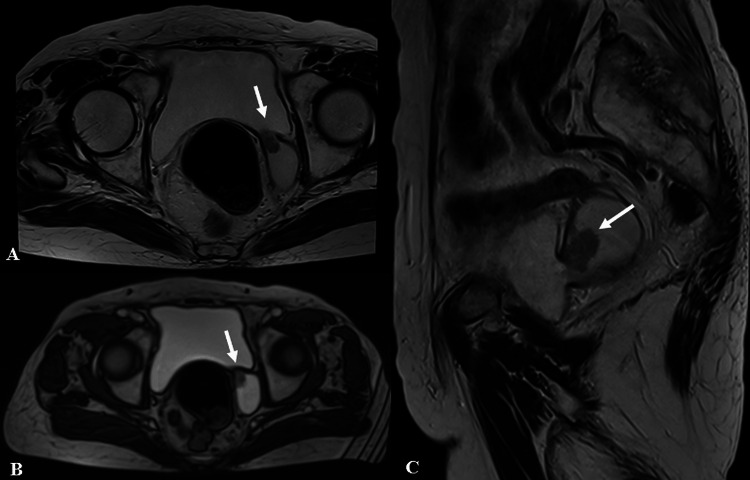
Radiographic finding: A 2.7 x 2.1 cm papillary lesion (white arrows) on magnetic resonance urography imaging of the left posterolateral bladder wall inside a diverticulum with intermediate signal intensity (A and B show the axial view while C shows the sagittal view).
